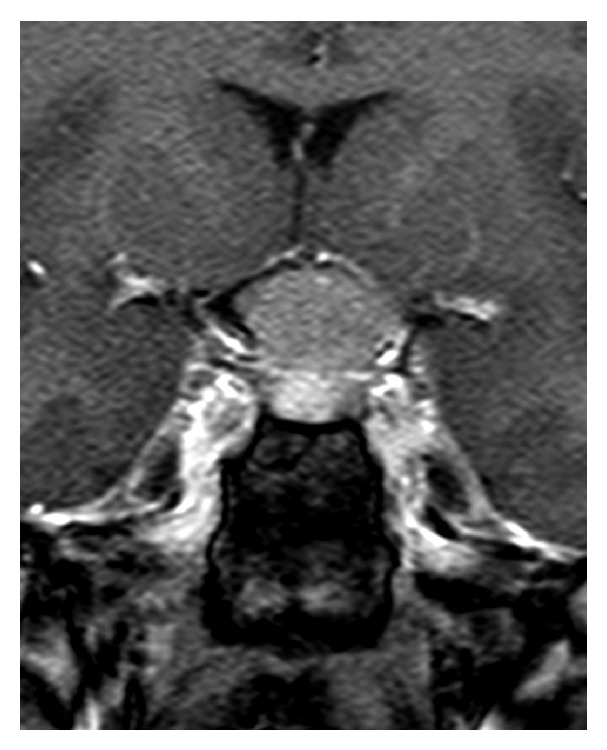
T1-weighted gadolinium-enhanced MRI revealed a tuberculum sellae meningioma measuring approximately 1.7 cm × 1.9 cm × 1.3 cm.
